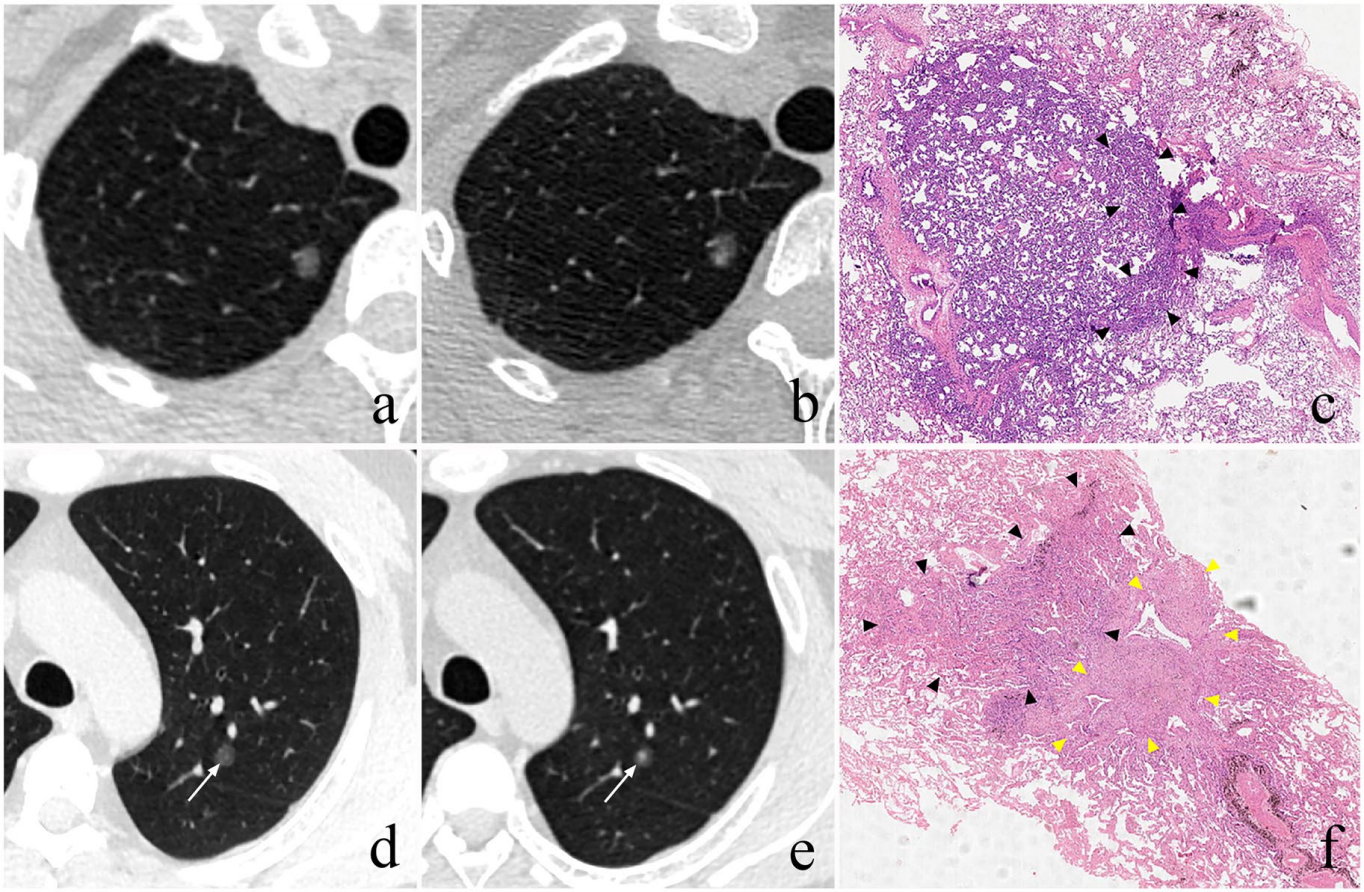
Representative cases demonstrating pathological characteristics of SSNs with attenuation growth only. (a–c) A 40-year-old female has a pGGN (diameter, 7.3 mm; volume, 171 mm³; CT value, −587 HU) located in the RUL. On follow-up CT (interval: 9.3 months), it shows an increase in CT value to −534 HU, but no significant growth in diameter (7.4 mm) and volume (184 mm³). After operation, it is confirmed as AIS, accompanied by alveolar collapse (black arrowheads). (d–f) A 38-year-old male has a pGGN (diameter, 7.2 mm; volume, 176 mm³; CT value, −696 HU) located in the LUL (white arrow). On follow-up CT (interval: 34.3 months), it shows CT attenuation increasing to −643 HU, but no significant growth in diameter (7.2 mm) and volume (179 mm³). After operation, it is confirmed as AIS, accompanied by alveolar collapse (black arrowheads) and fibrous tissue proliferation (yellow arrowheads). SSNs, subsolid nodules; pGGN, pure ground-glass nodule; CT, computed tomography; HU, Hounsfield unit; RUL, right upper lobe; LUL, left upper lobe; AIS, adenocarcinoma *in situ.*
